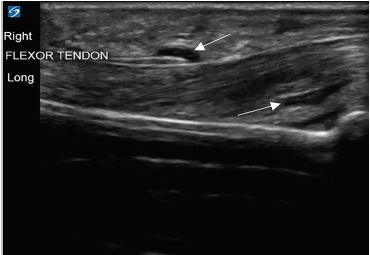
Longitudinal ultrasound view of the right middle finger. The image displays the striated flexor tendon with anechoic fluid (white arrows) above and below the tendon.
